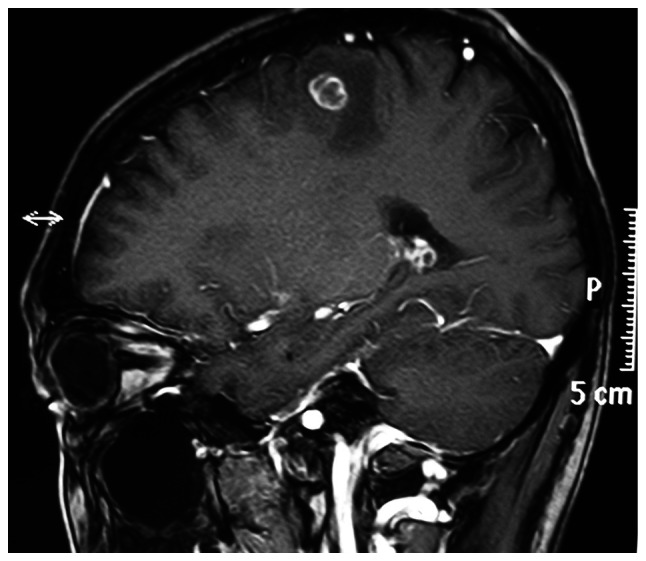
T1-weighted magnetic resonance imaging (MRI) section through the brain shows single brain metastasis from lung disease.
